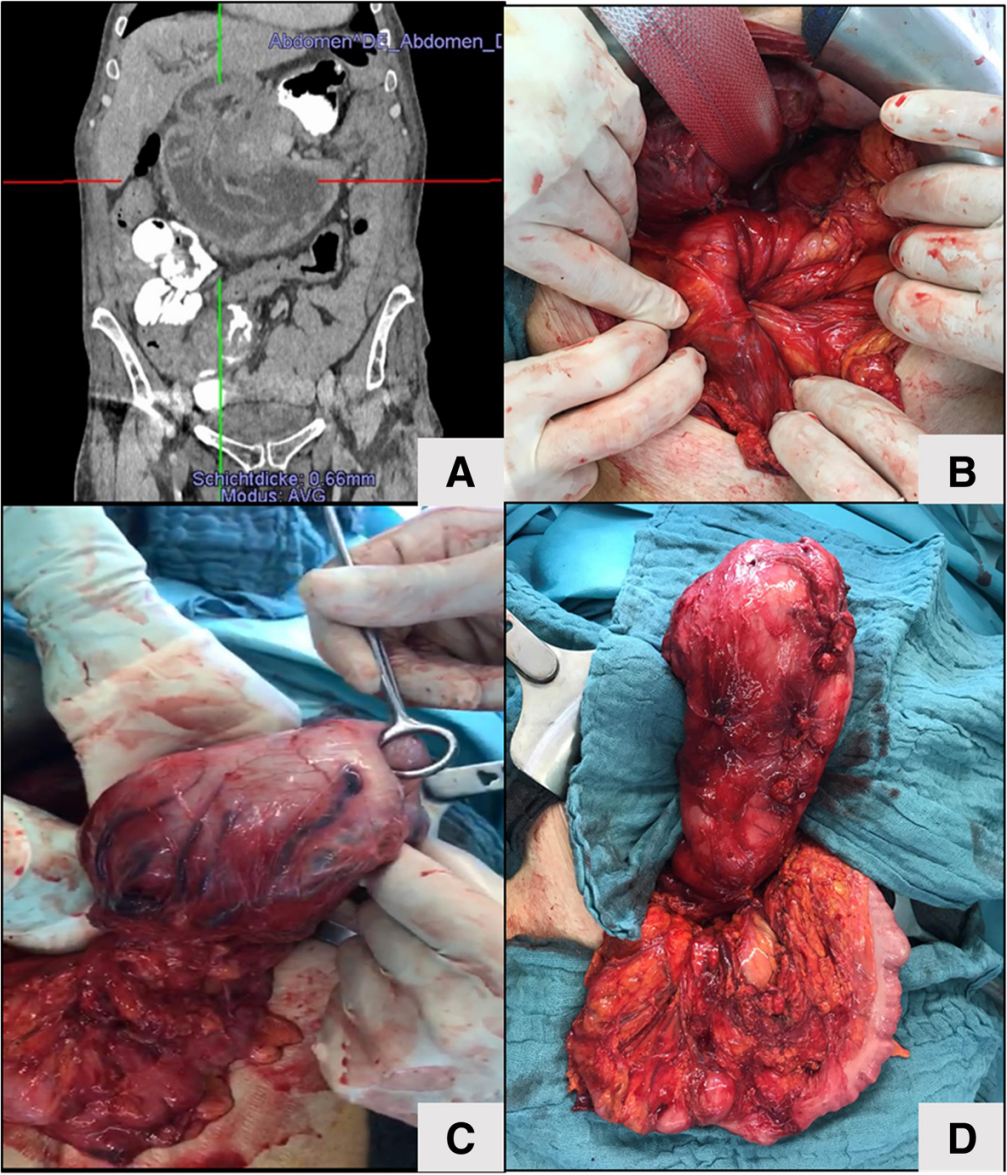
a Coronal cross section CT with oral contrastation with a target sign. b Intraoperative image of the negative lumen of the intussuscepted remnant stomach. c Blunt manual reposition of the remnant stomach. d Fully repositioned remnant stomach (top) with the transverse colon (bottom) as a size comparison
